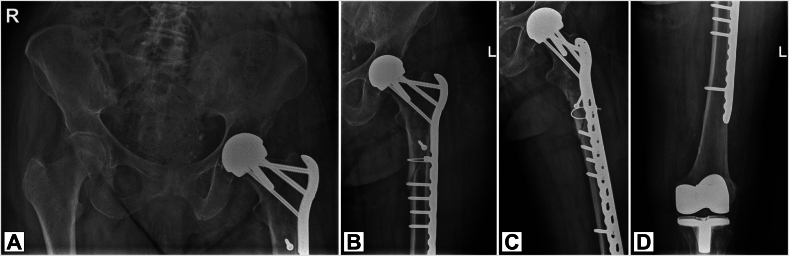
Postoperative radiographs, four months after reduction and fixation of subtrochanteric femoral fracture with a Locking Compression Plate, cerclage wires and multiple cortical screws, showing signs of callus formation. A: Anterior-Posterior (AP) X-ray of pelvis. B: AP X-ray of proximal left femur. C: Lauenstein X-ray of left femur. D: AP X-ray of distal left femur.
